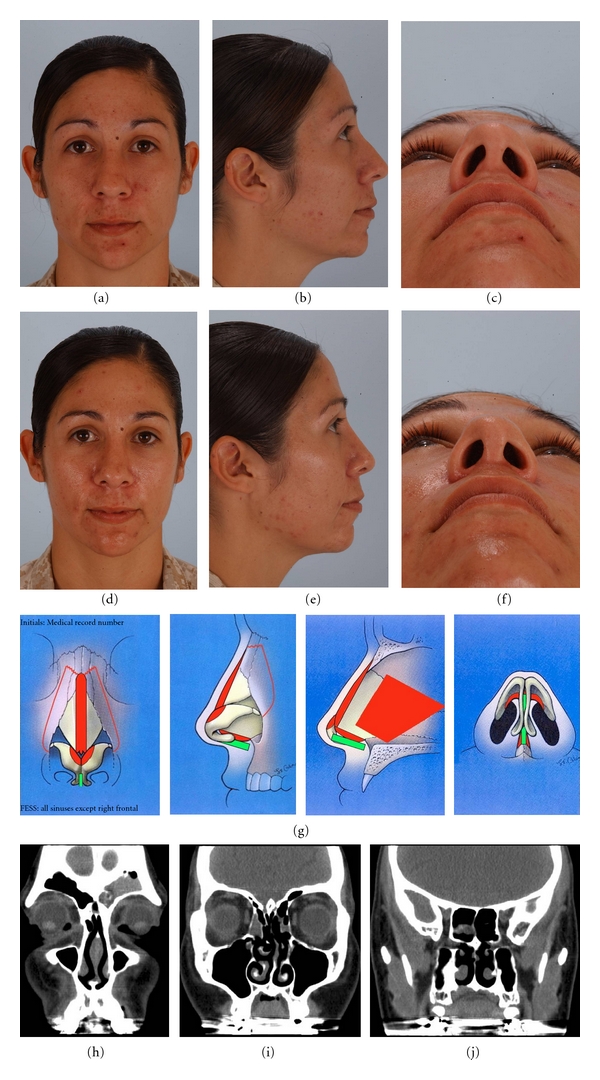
27-year-old female with chronic sinusitis who wanted a smaller more refined nose. Preoperative views: frontal (a), lateral (b), submental (c). One-year Postoperative views: frontal (d), lateral (e), submental (f). Intraoperative rhinoplasty worksheet indicating septoplasty, profile alignment, osteotomies, cephalic strip removal, and osteotomies (g). Preoperative serial coronal CT scans showing extensive sinus disease (h, i, j).
